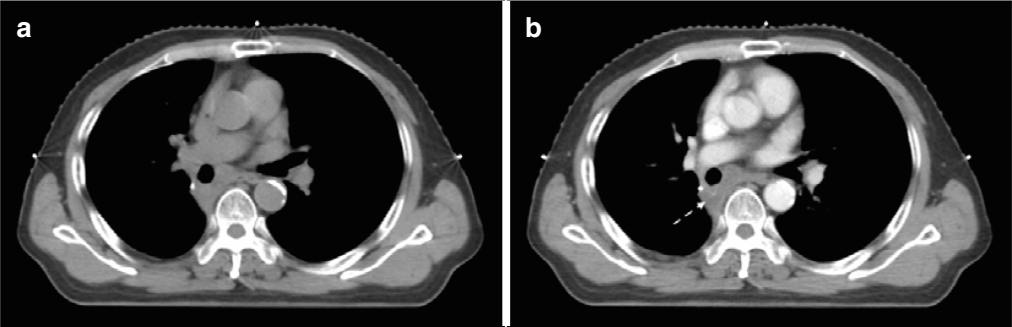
The Hounsfield units (HUs) of hilar lymph nodes in the plain 3D-CT (a) and CE-4D-CT (b) were 24 ± 18 and 37 ± 19, respectively. Lymph nodes showed low enhancement, but peripheral vessels were highly enhanced, and the lymph nodes (*dotted arrow*) were clearly identified
